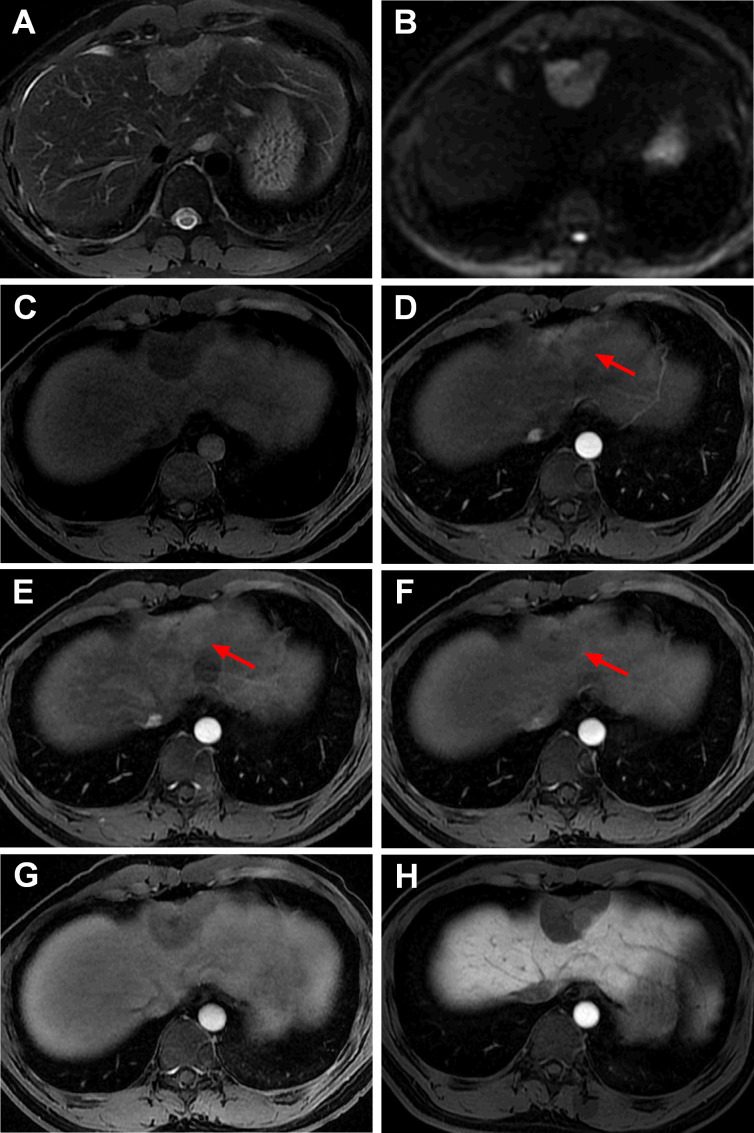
MRI images of IHS in case one. The segment II/IV mass was slightly hyperintense on the *T*
